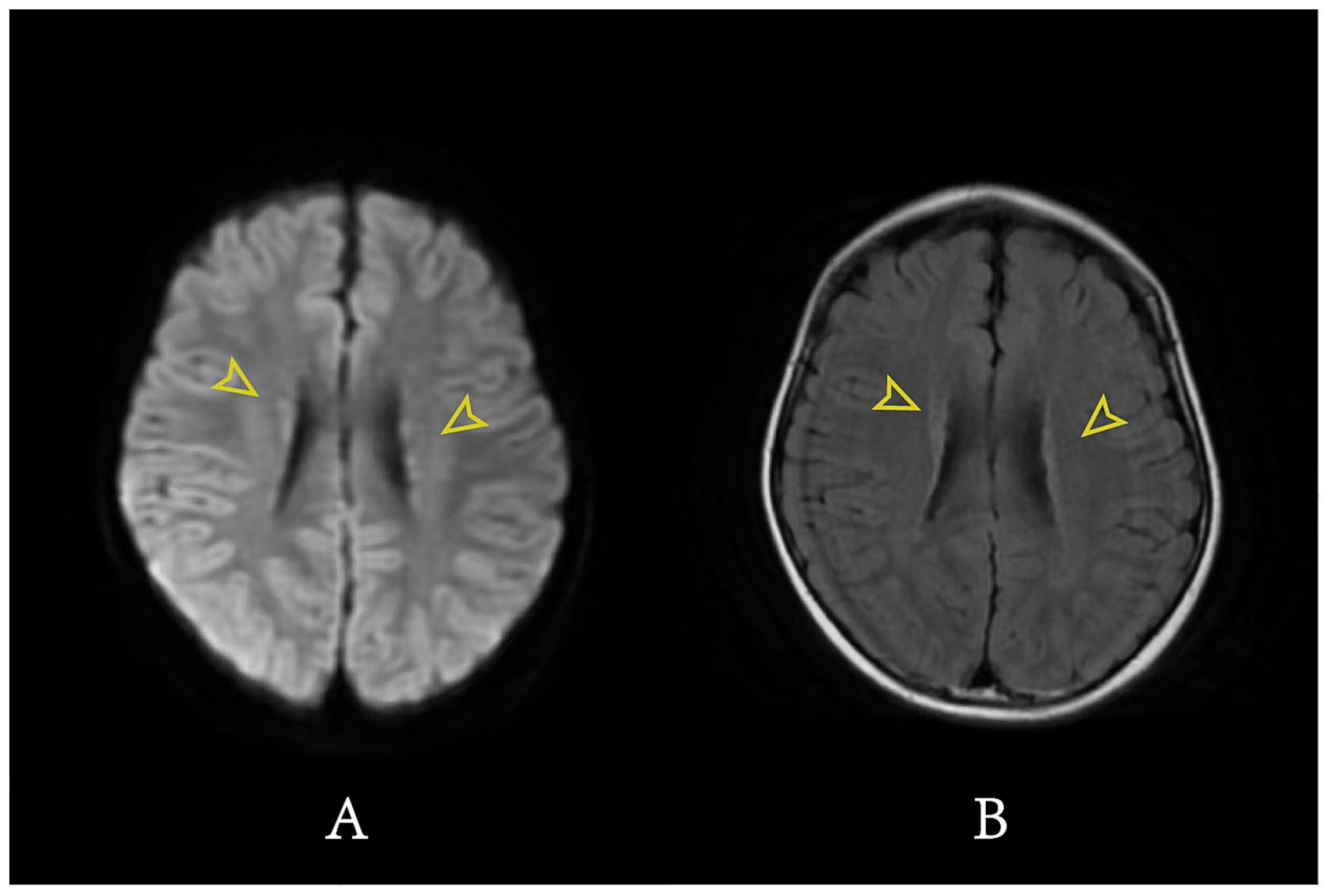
MRI demonstrates bilateral periventricular gray matter heterotopia. (A) DWI MRI; (B) T2 Flair MRI; Yellow arrows: bilateral periventricular gray matter heterotopia.
